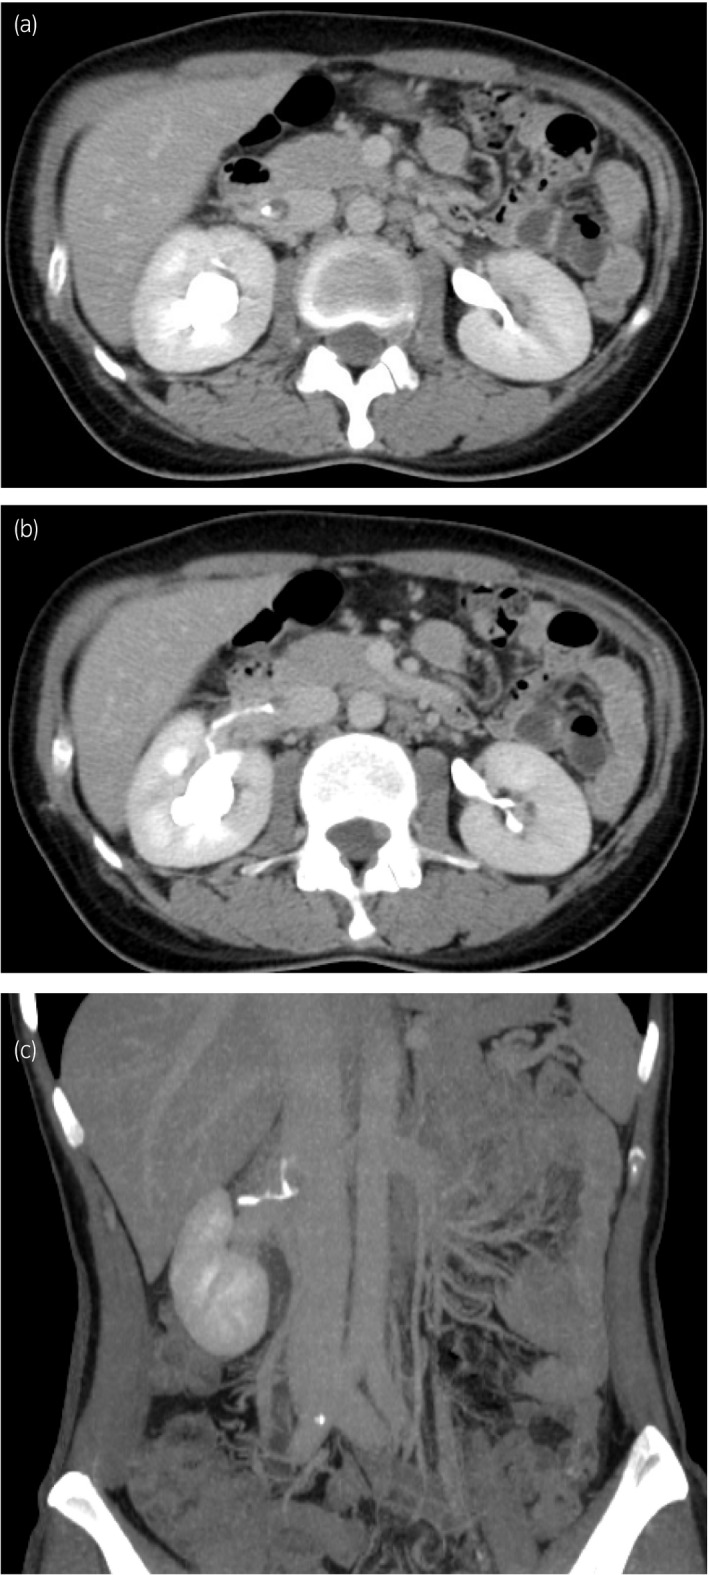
Axial images (a,b) and a coronal reconstructive image (c) during the excretory phase of contrast‐enhanced CT before ureteroneocystostomy. (a) The contrast medium flowed into the low‐density lesion in the IVC, and the renal pelvis was filled with contrast medium. (b) A thin, meandering backflow of the contrast medium from the right renal pelvis through the renal vein. (c) Contrast medium going cephalad along the IVC.
